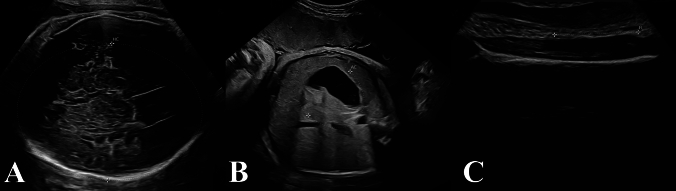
Ultrasound images showing wrong AI measurements leading to underestimation of A the head circumference, B the abdominal circumference, and C the femur length
